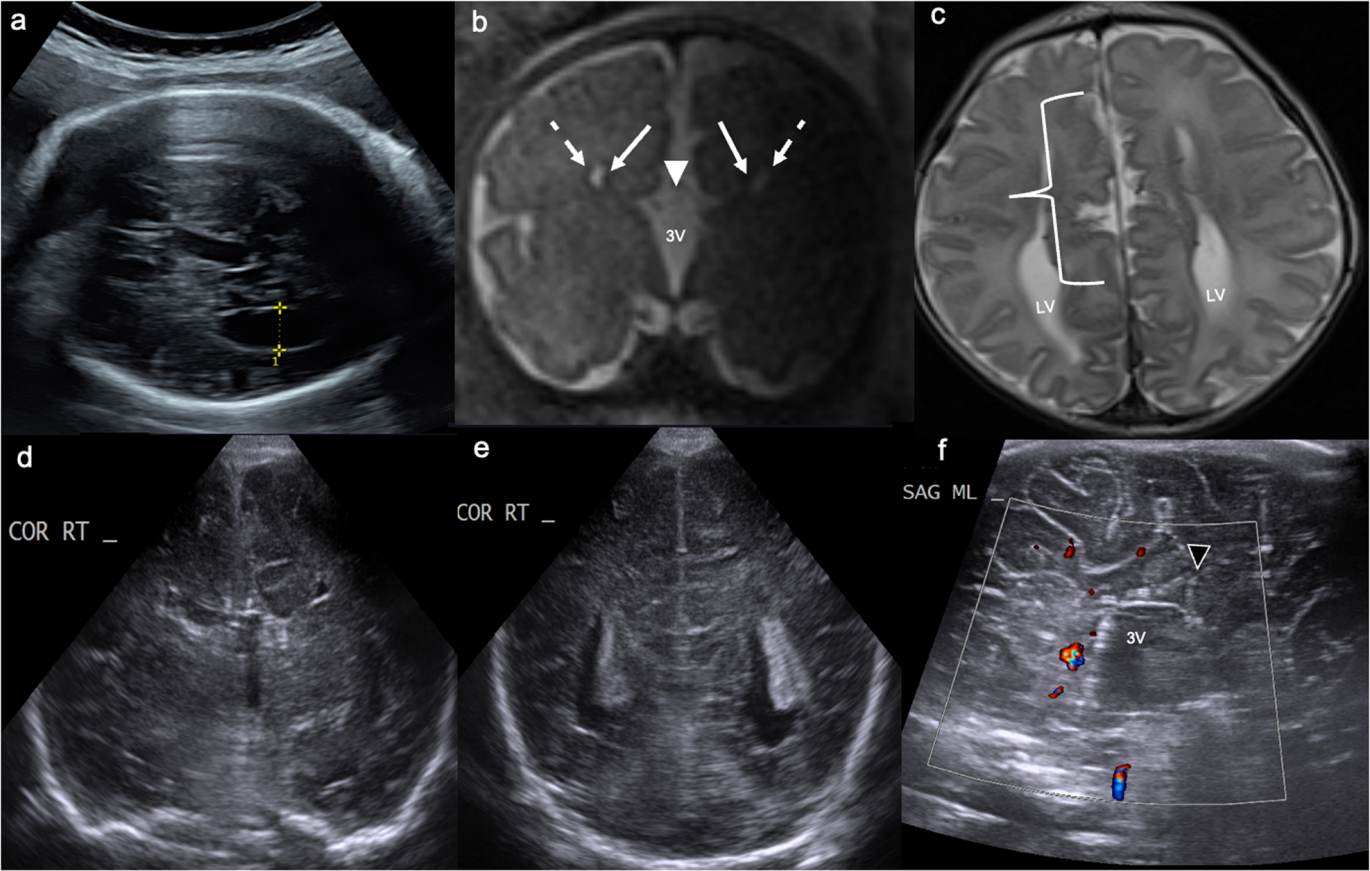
Callosal agenesis. a Antenatal ultrasound demonstrates the absence of the septum pellucidum and enlargement of the right lateral ventricular occipital horn (labelled 1). b Coronal T2W fetal MRI confirms the absent septum pellucidum, with medially indented (solid arrows) and laterally convex (dashed arrows) frontal horns, and a high-riding third ventricle (3V) extending to the interhemispheric fissure (white arrowhead). c Axial T2WI MRI neonatally shows symmetrical enlargement of the lateral ventricular (LV) occipital horns, e.g., colpocephaly, which have a parallel “racing car” configuration; right frontal polymicrogyria is also noted (bracket). d Coronal ultrasound at term echoes the fetal MRI findings outlined in b above. e It also confirms colpocephaly and parallel orientation of the lateral ventricles. f Midline sagittal ultrasound demonstrates radially oriented cingulate gyri (black arrowhead) extending to the 3rd ventricle (3V), and no detectable corpus callosum or pericallosal artery
